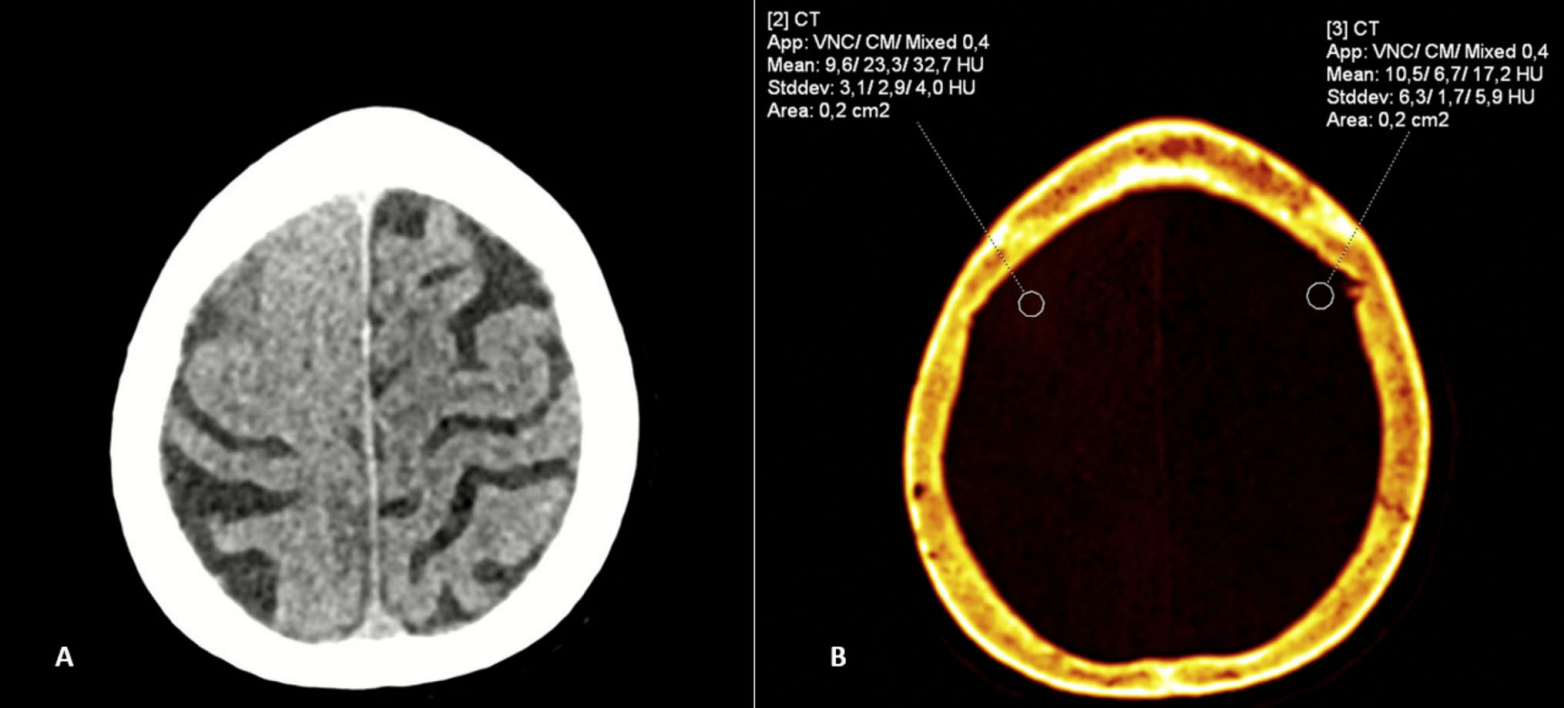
After a percutaneous coronary intervention, an 85 y-o woman presented mild left hemiparesis. Virtual MonoEnergetic (VME, 80 and 140 kV scans) image shows diffuse subarachnoid hyperdensity over the right hemisphere. Virtual non-contrast/contrast media (VNC/CM) image at the same level shows that hyperdensity (32.7 HU) is due to contrast extravasation (CM: 23.3 HU) and not to subarachnoid hemorrhage (VNC: 9.6 HU). Imaging by IRCCS Policlinico San Donato, Milan, Italy, by Siemens Somatom Definition Flash 256 slices scanner and Syngo CT dual-energy tool
